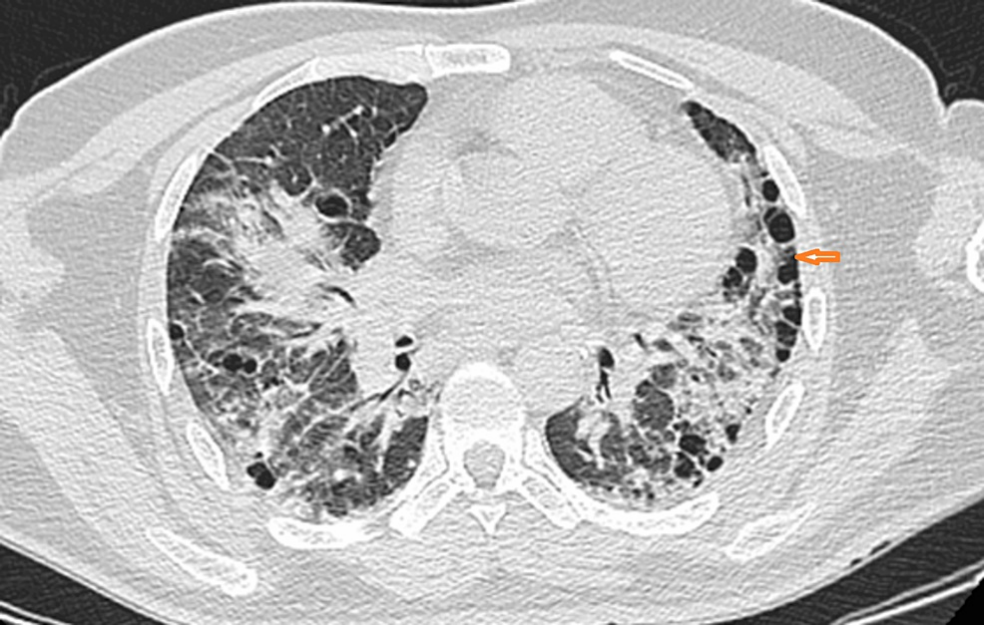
Axial CT scans of the chest with lung window settings showing honeycombing.
